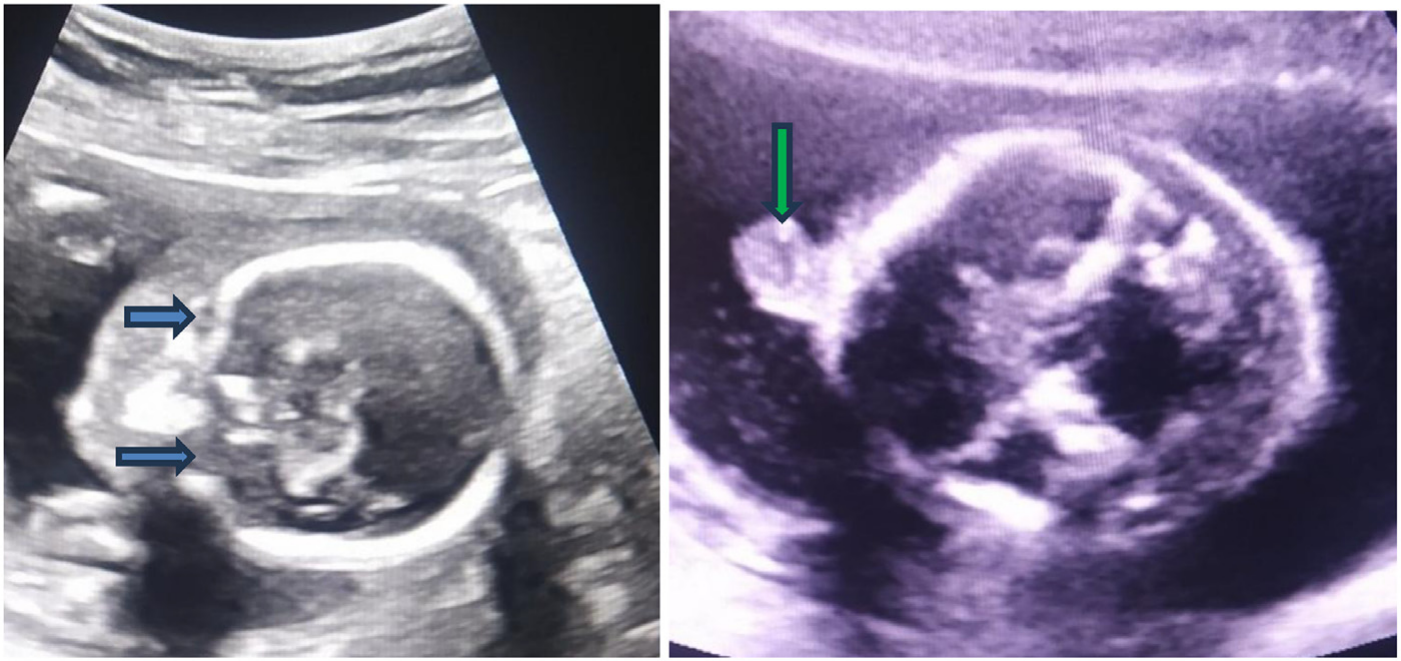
Ultrasound of the fetal face at 19 weeks gestation (Philips IU22, convex probe of 1-7 MHz). (A, the left image, coronal plane) Axial plane view showing the absence of normal orbital structures; the ocular globes are not visualized within the expected regions (blue arrows). (B, the right image, axial plane) A midline, oval, partly cystic structure is seen protruding from the forehead region (green arrow), representing a proboscis. A proboscis with absent orbits is characteristic of cyclopia associated.
